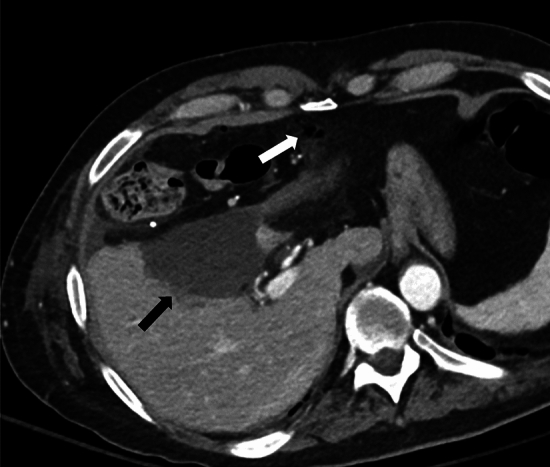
A 59-year-old who underwent cholecystectomy for acute on chronic cholecystitis with biloma secondary to bile leak. A postoperative collection is seen in the surgical bed (black arrow). Postsurgical pneumoperitoneum is also seen (white arrow). CT-guided aspiration revealed bilious contents. ERCP demonstrated a low-grade leak from the cystic duct, which was managed with biliary sphincterotomy and stent placement
